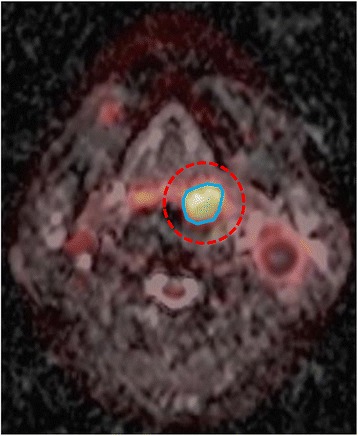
Measuring methods of simultaneous ^18^F-FDG PET/MRI parameters. As an initial step, the regional PET and ADC map were integrated. For the acquisition of PET parameters (SUVmax, MTV, and TLG), a spherical VOI (dashed red circle) was drawn to include the whole tumor. An isoactivity contour (solid blue circle) was automatically drawn by setting a threshold of SUV 2.5, and PET parameters were acquired. For an MRI parameter, a VOI was drawn along the isoactivity contour of PET, and the ADCmean of the tumor was evaluated
